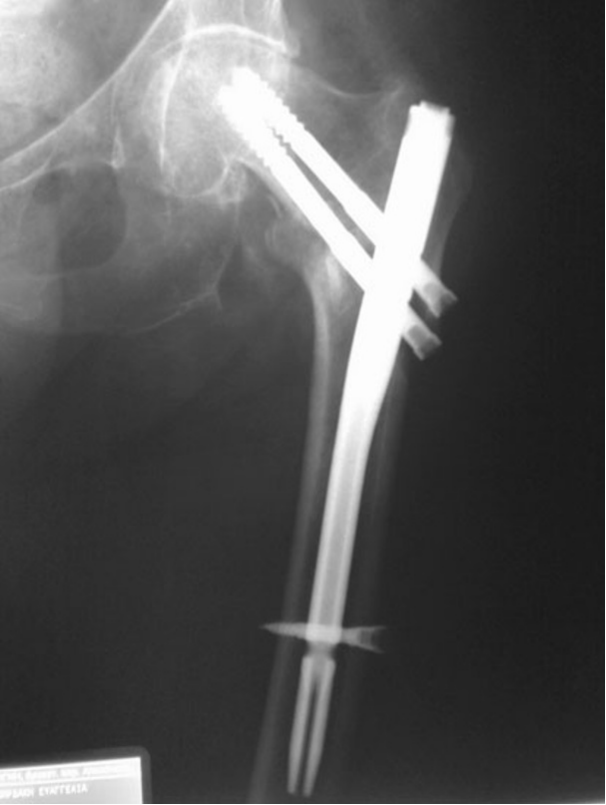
X-ray showing a case in which distal locking screws were missed. The screws left in place and the postoperative protocol were followed as usual without any further complication
